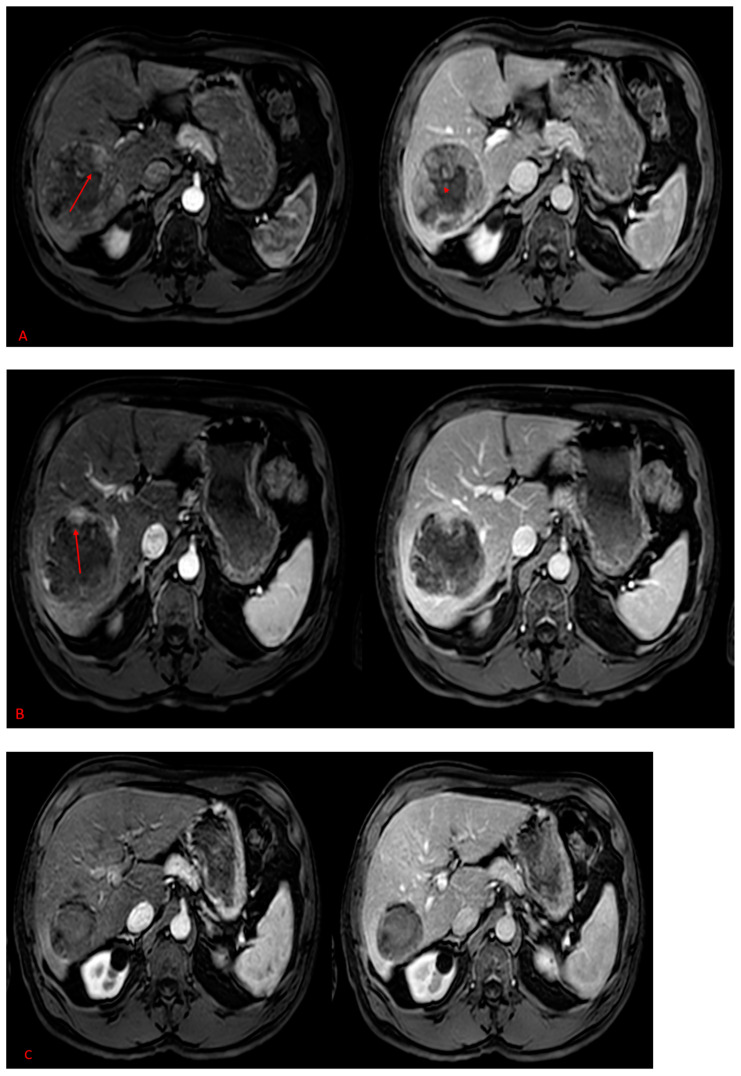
(A–C) A 73-year-old male with HCC in the right lobe, MRI images centered at the level of the celiac trunk, in different phases; arterial phase in the left and port venous phase in the right. (A) The initial MRI shows a large heterogeneous hypervascular tumor in the periphery with central necrosis. (B) After 2 TACE sessions, failure of the treatment, with persistence of enhancing viable remnant tissue as marked by the red arrow, compatible with residual disease. (C) MRI after six months of SIRT demonstrating a complete viable tumor regression.
